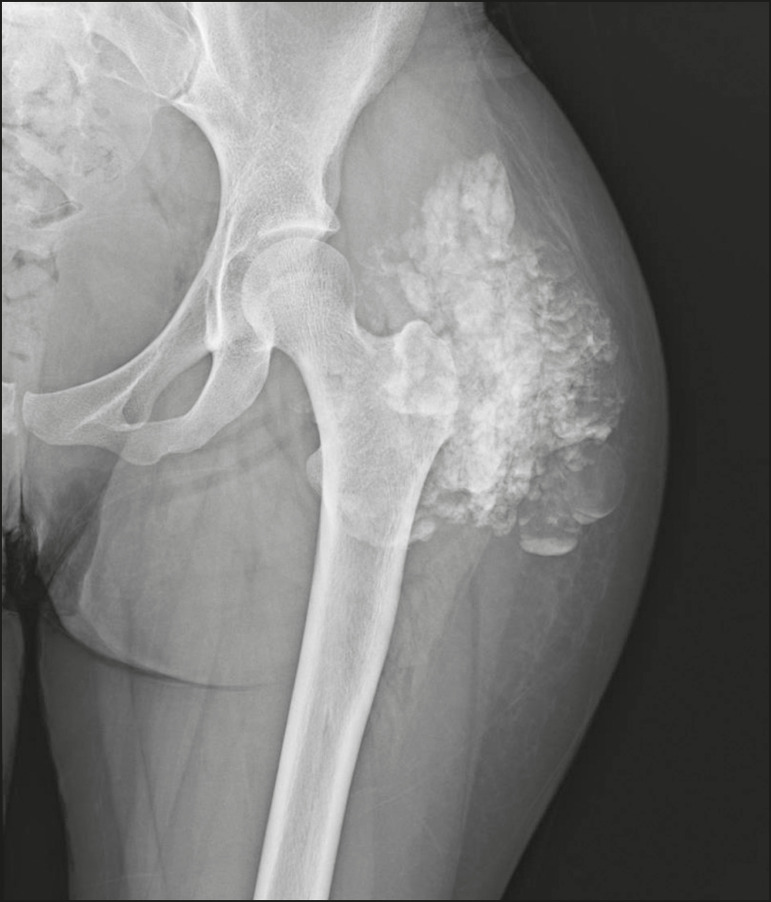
Tumoral calcinosis. Frontal X-ray of the hip showing a calcified peri- calcifications occurring in the thumbs and index fingers. articular mass with lobulated contours and a multicystic appearance in the trochanteric bursa. A fluid-calcium level (the sedimentation sign) can be seen in the cysts.
